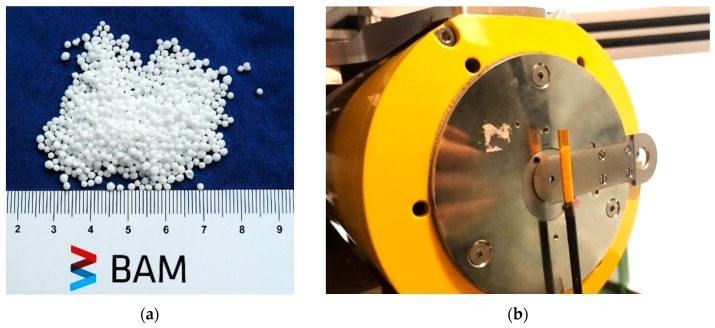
Photography of ammonium nitrate (AN) prills type E1: (a) loose AN prills; (b) AN prills paced into a polyimide tube glued onto a carbon fibre rod, prior to X-ray computed tomography (XCT) scanning.
